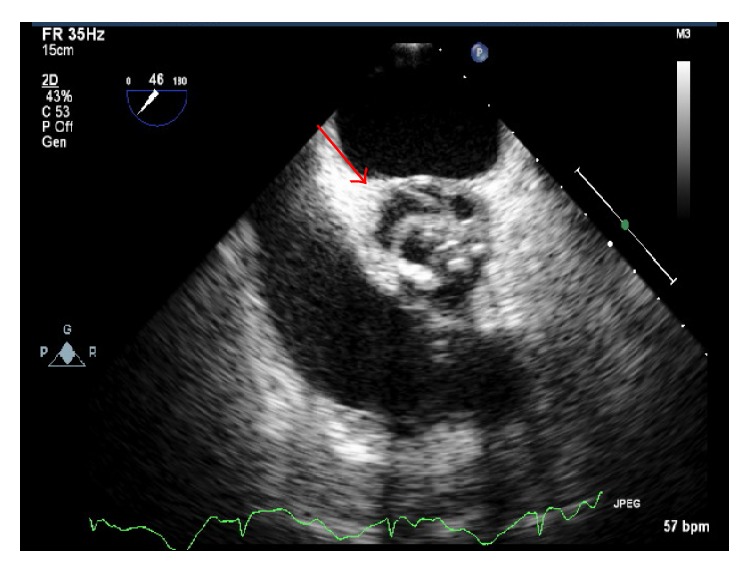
Transesophageal echocardiogram at midesophageal inflow/outflow tract revealing subannular abscess, in diastole.
